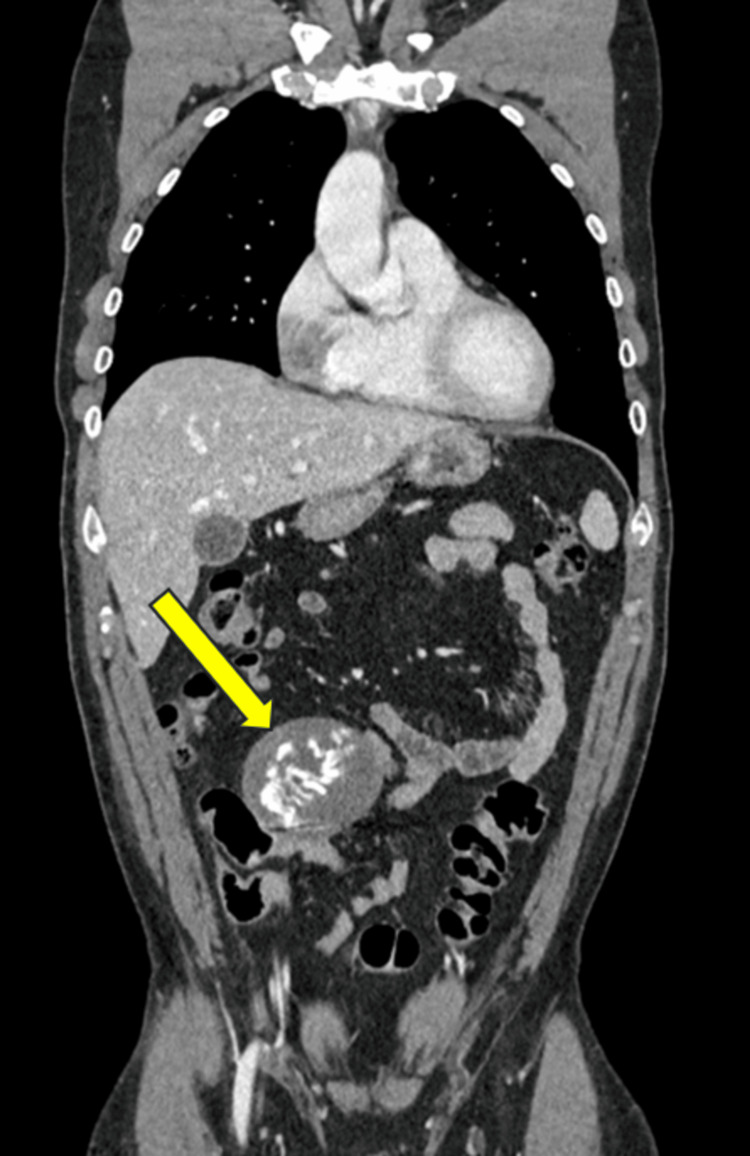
CT scan shows the gossypiboma in the right iliac fossa in coronal view. The yellow arrow indicates the gossypiboma.
